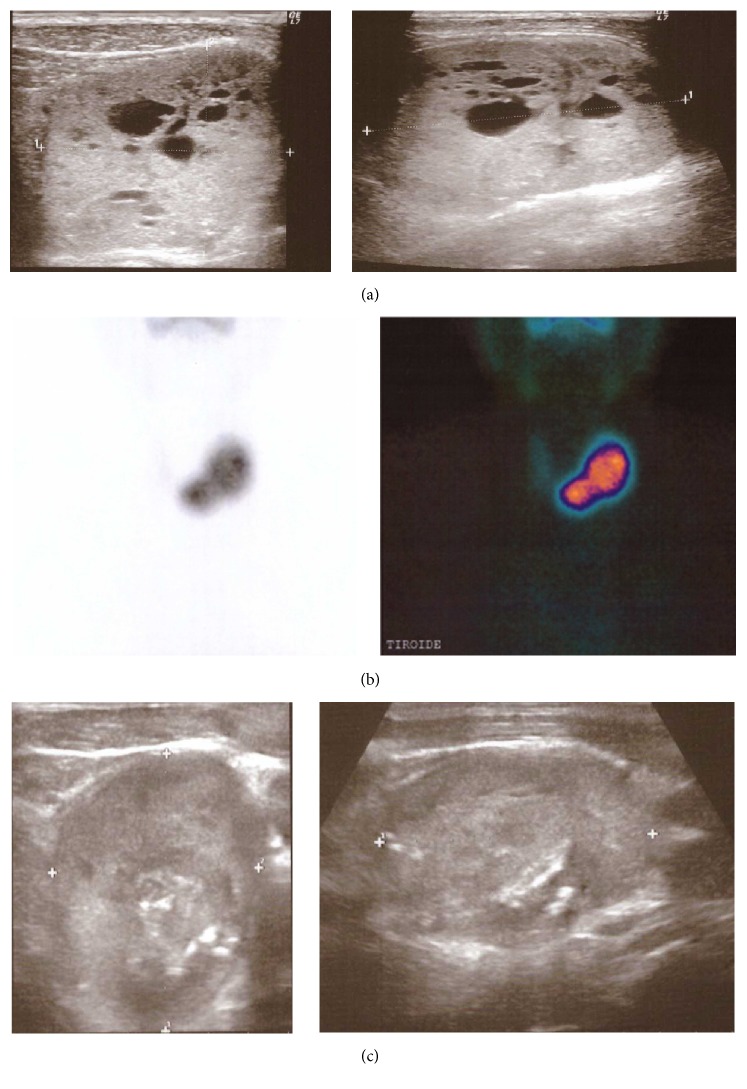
(a) Ultrasound of left lobe nodule at baseline; (b) thyroid scan (99mTc-pertechnetate); and (c) ultrasound of left lobe nodule after LAT + 131I therapy.
